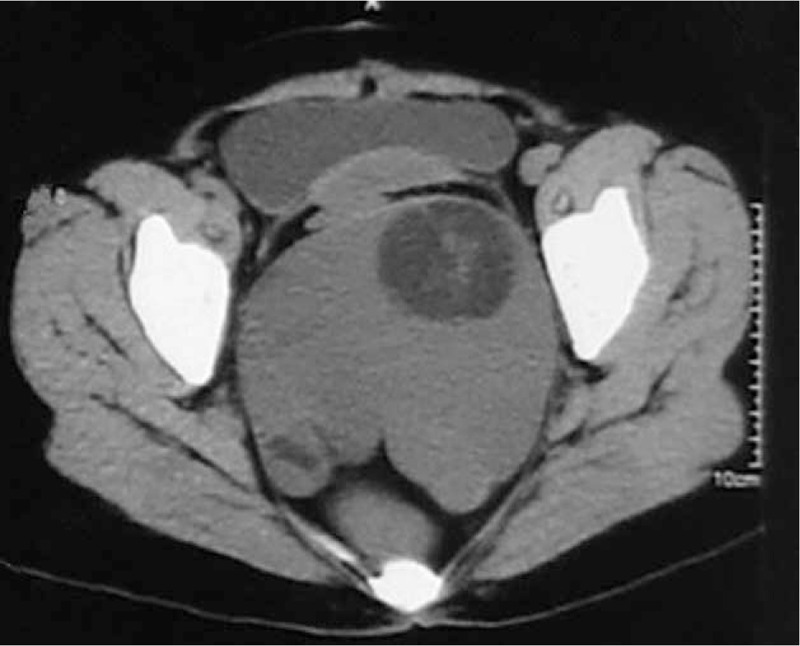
The CT image took in October 2014, 14 cm in size.
